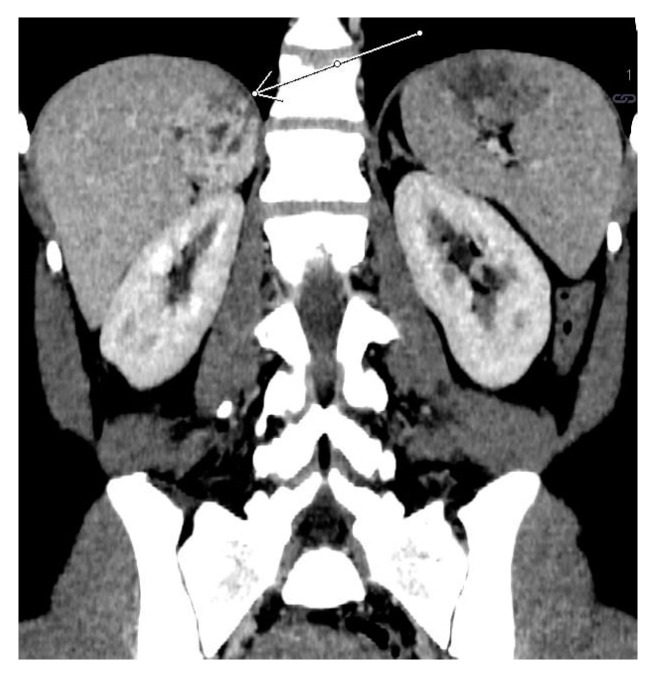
Adrenal cortical carcinoma. Abdomen CT, longitudinal cross-section.
